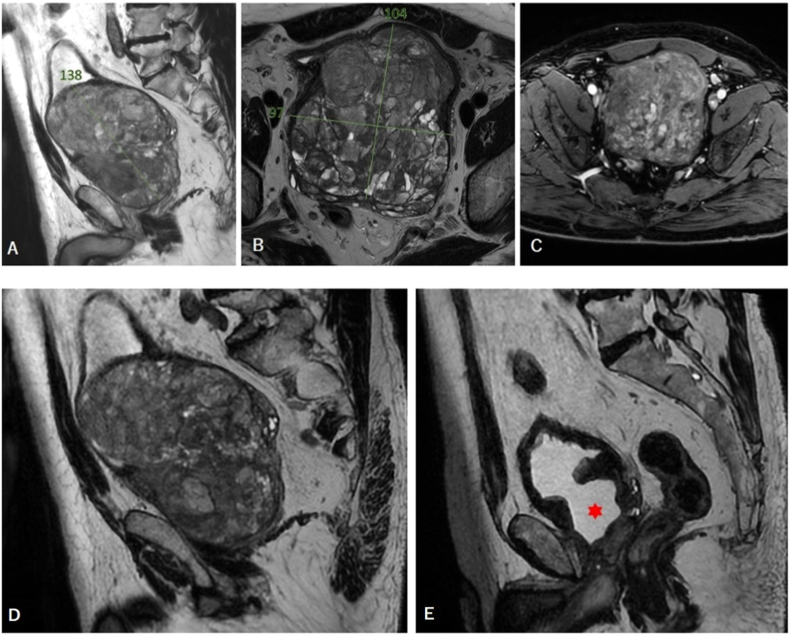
Sagittal T2 (A), axial T2 (B) and axial T1 with fat suppression (C) cuts on a pre-operative multiparametric MRI of the prostate. A pre- (D) and post-operative (E) prostate MRI-based comparison on a sagittal view showing the empty prostatic fossa (red star) at 2 months after HoLEP. (For interpretation of the references to colour in this figure legend, the reader is referred to the Web version of this article.)
